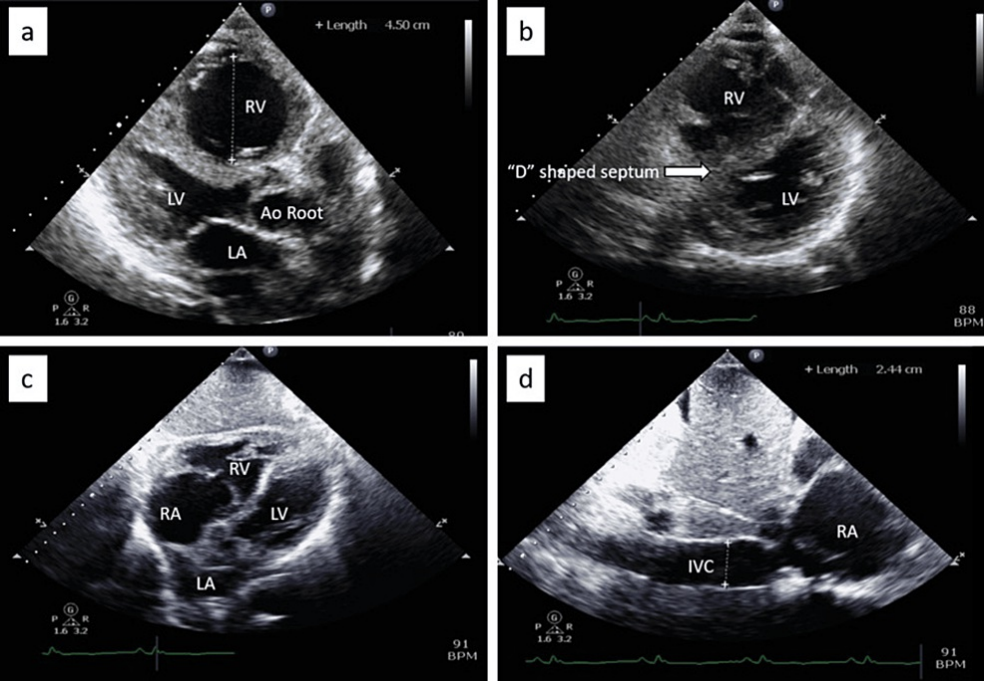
Transthoracic echocardiography (TTE) images showing the following: (a) parasternal long axis view showing a severely dilated RV measuring 4.5cm, (b) parasternal short axis view showing a “D” shaped interventricular septum sign suggestive of RV pressure and volume overload, (c) subcostal four-chamber view showing dilated RV and RA, and (d) subcostal view showing dilatation of the IVC at 2.44cm. RV: Right ventricle; LV: left ventricle; LA: left atrium; Ao root: aortic root; RA: right atrium; LA: left atrium; IVC: inferior vena cava
